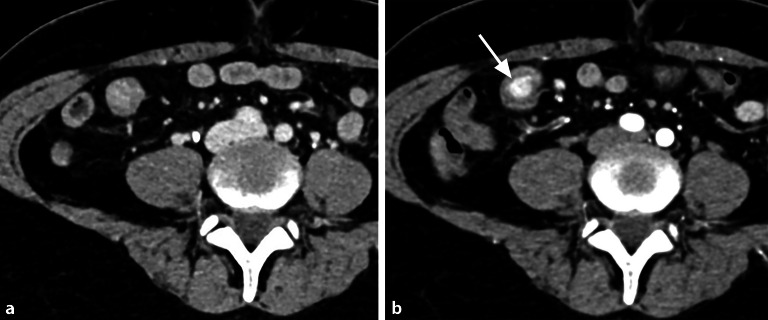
Intraluminaler Tumor des Dünndarms (neuroendokriner Tumor, NET), der in der venösen Computertomographie (CT; a) nicht eindeutig, in der arteriellen Phase (b) jedoch als hypervaskularisierte intraluminale Läsion deutlich abgrenzbar ist (*Pfeil*)
